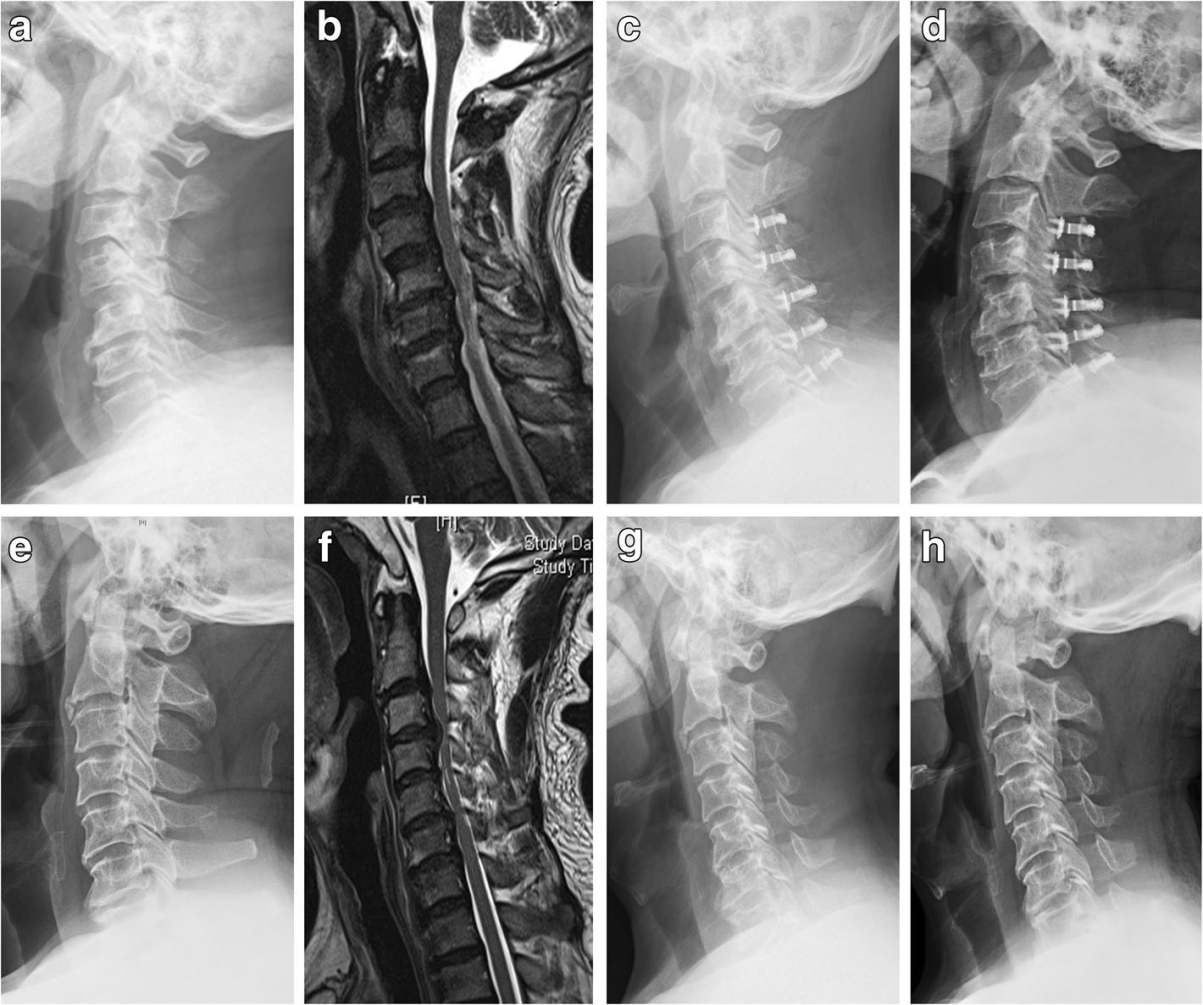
Comparison of cervical sagittal imaging parameters between two surgical approaches. a–d PODL secured with titanium miniplates (centerpiece). a Preoperative lateral cervical X-ray showed cervical degenerative and multi-segment spinal stenosis, with C2-7 SVA of 10.6 mm. b Preoperative cervical MRI showed multi-segment spinal compression at C3-7 segments. c At 1 month after surgery, lateral cervical X-ray showed that there was apparent increase of cervical canal width, with significant cervical anteversion and C2-7 SVA of 29.6 mm. d At 2 years after surgery, lateral cervical X-ray indicated good position of the titanium miniplates, and there was significant improvement of cervical anteversion, with the C2-7 SVA of 12.1 mm. e–h PODL secured with silk anchors. e Preoperative lateral cervical X-ray showed cervical degenerative and multi-segment spinal stenosis, with C2-7 SVA of 20.5 mm. f Preoperative cervical MRI showed multi-segment spinal compression at C3-7 segments. g At 1 month after surgery, lateral cervical X-ray showed that there was apparent increase of cervical canal width, with significant cervical anteversion and C2-7 SVA of 33.6 mm. h At 2 years after surgery, lateral cervical X-ray indicated good position of the anchors, and there was mild improvement of anteversion, with the C2-7 SVA of 29.1 mm
